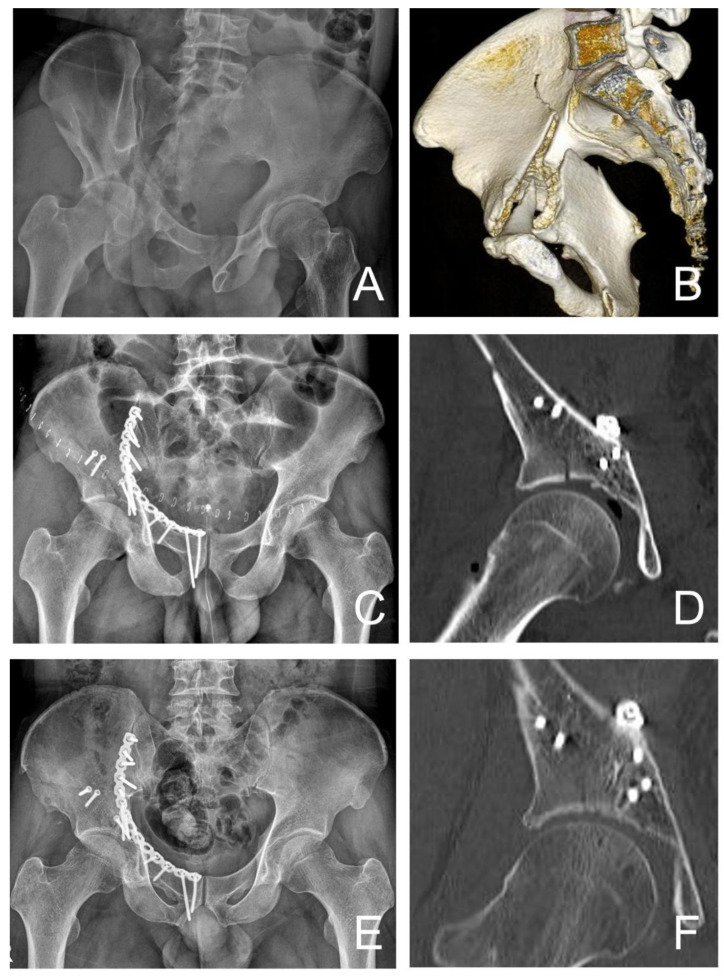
A 41-year-old man underwent indirect fixation with a suprapectineal plate. (A) Radiograph shows both-column fracture with 30 mm displacement. (B) Three-dimensional computed tomography (CT) scan shows both-column fracture with quadrilateral plate displacement. (C) Indirect fixation with suprapectineal plate is performed. (D) CT scan shows congruent reduction. (E) Radiograph obtained 41 months after surgery shows union at the fracture site and good grade with Matta’s outcome grading. (F) CT scan also shows mild joint space narrowing, but no sign of osteophyte, subchondral sclerosis, and femoral head collapse. CT = computed tomography.
